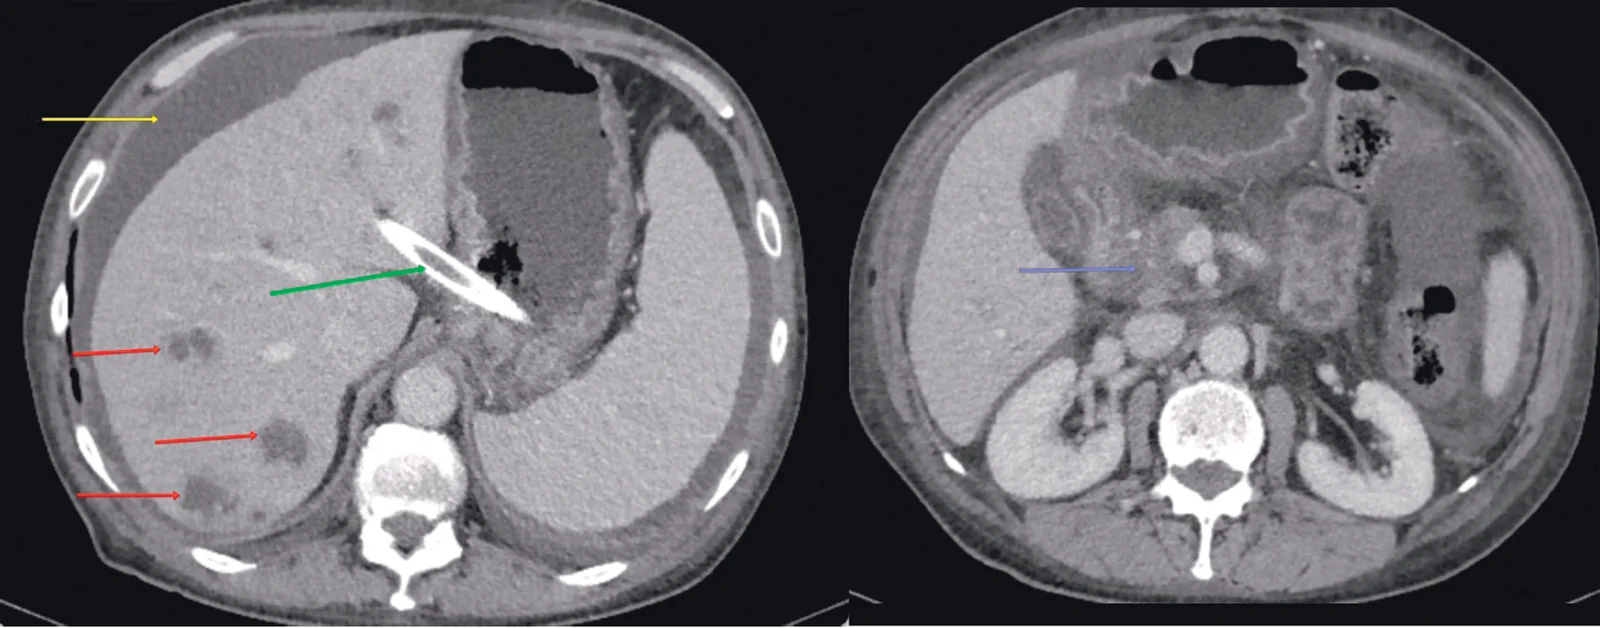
Left: CT scan showing the progression of the disease under
